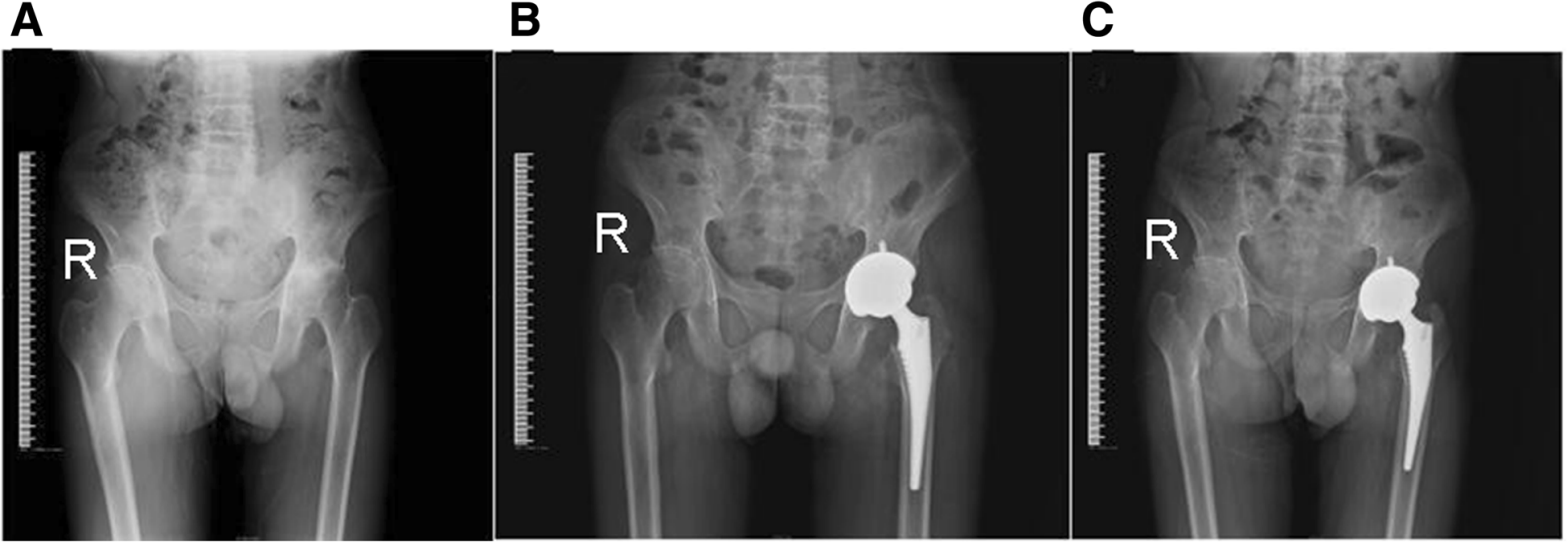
X-ray films of 2 typical cases before and after operation. a Male, 39 years old, preoperative pelvic X-ray film. b Male, 39 years old, postoperative 2 days, the pelvic normal X-ray. c Male, 39 years old, postoperative 5 year pelvic X-ray film
